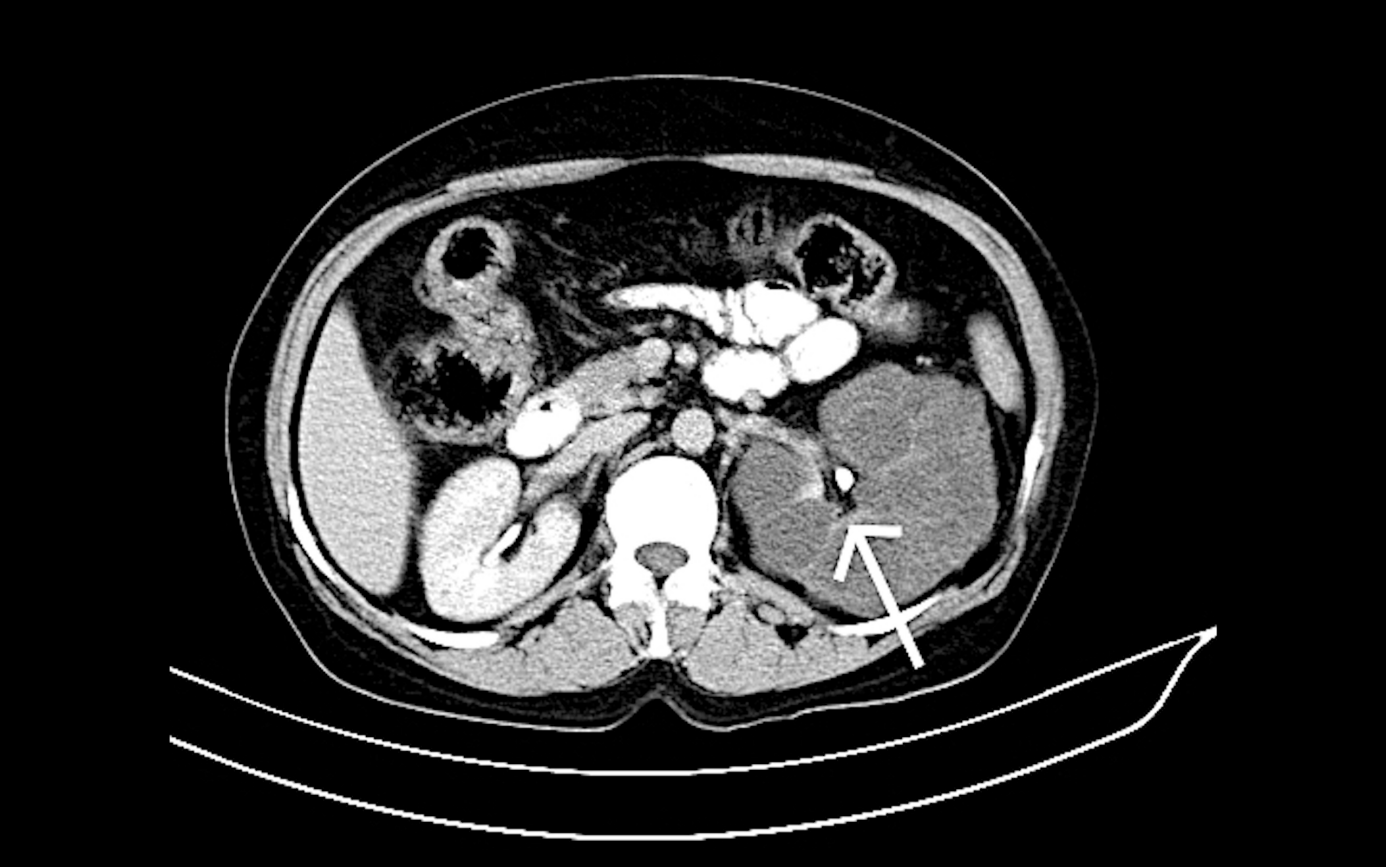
A 39-year-old male patient with complaints of left flank pain and abdominal distension. Intravenous contrast-enhanced axial computed tomography scan section of the left kidney shows multiple simple unenhanced hypodense cysts separated by contrast-enhanced thinned out residual renal tissue (white arrow).
